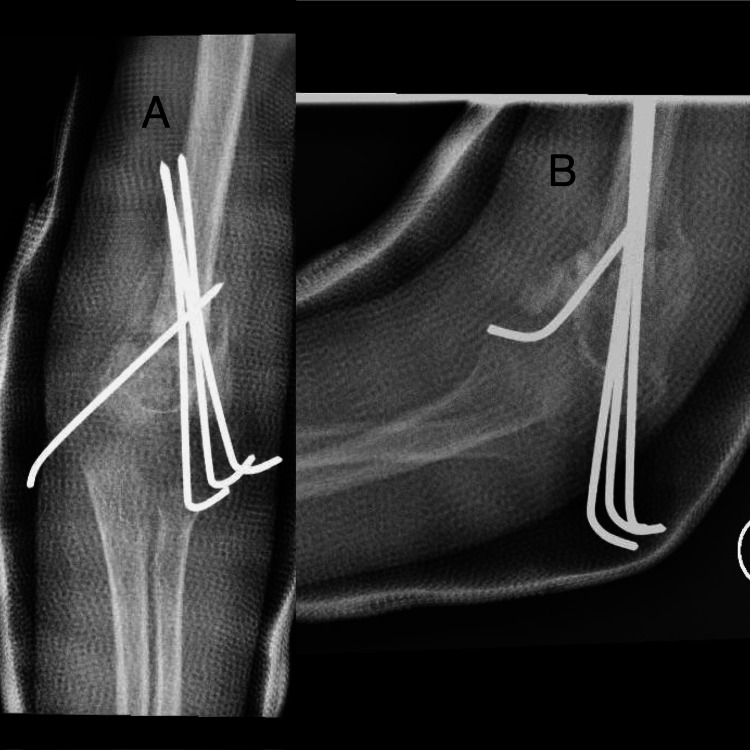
X-ray at the three-week follow-up (A) anteroposterior view; (B) lateral view
